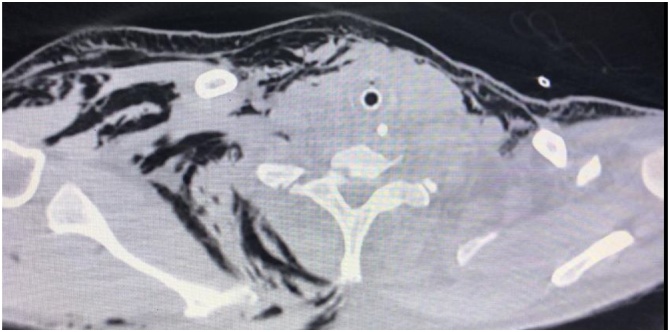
Thorax CT without contrast demonstrated subcutaneous emphysema after chest tube placement.
